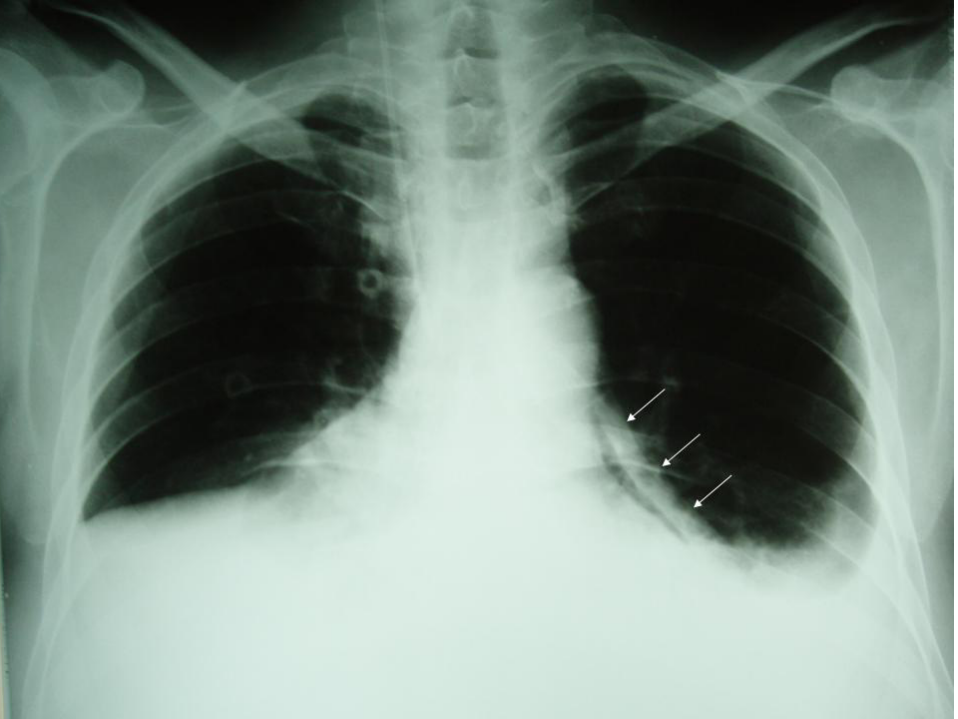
Plain chest radiograph demonstrating the presence of air in the left lateral pericardium (arrows) along with a small left pleural effusion.
